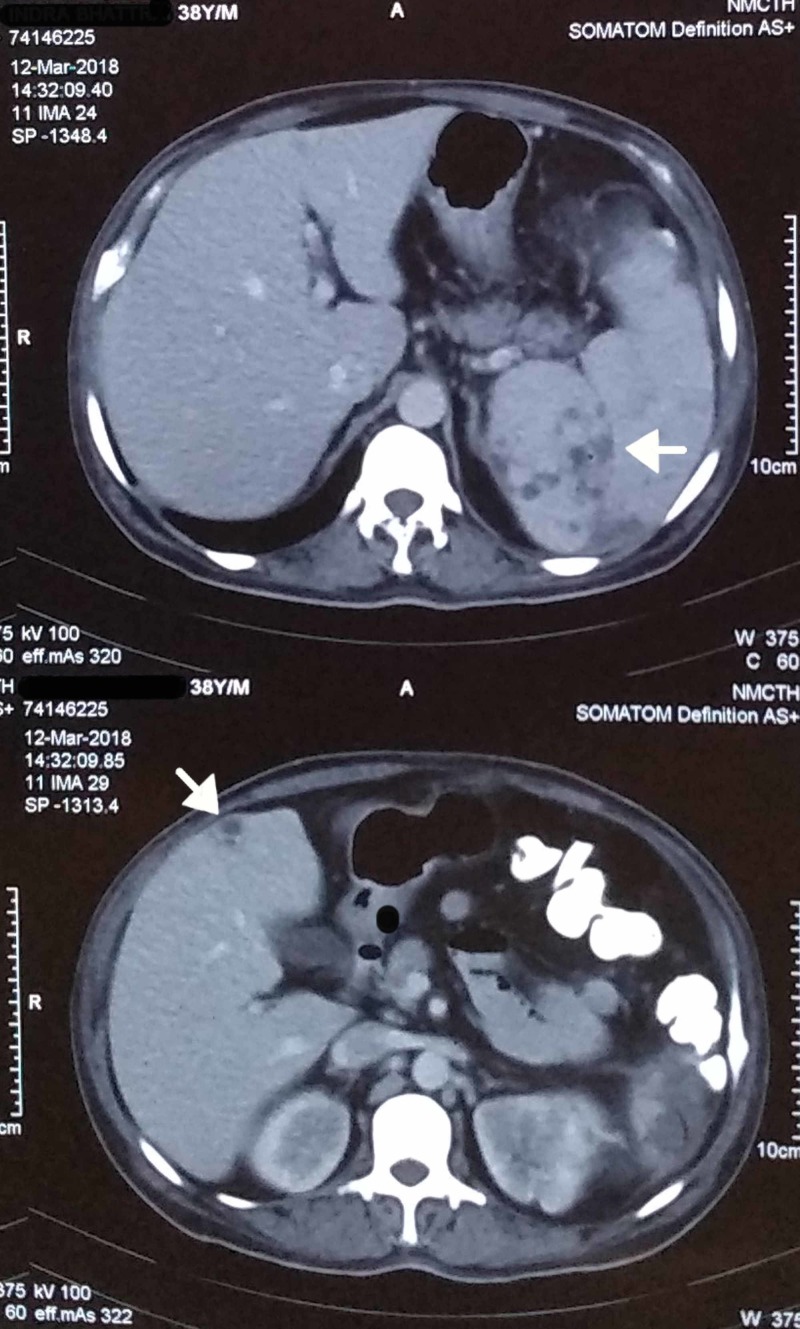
CECT of the abdomen showing hepatosplenomegaly with white arrows localizing ill-defined hypoechoic lesions in the liver and spleen, suggestive of abscesses. CECT, contrast-enhanced computed tomography
